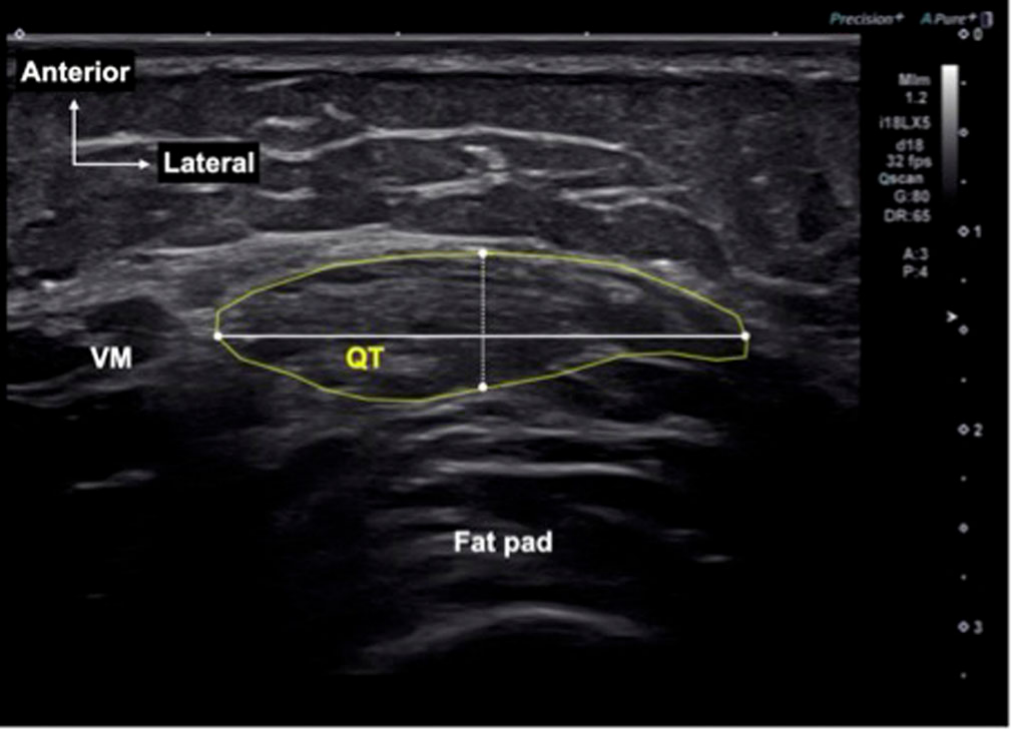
Measurement of anterior‐posterior thickness, medial‐lateral width, and cross‐sectional area (CSA) of the quadriceps tendon (QT) on magnetic resonance imaging. CSA (yellow line) was also measured at 15, 30, 45 and 60 mm proximal to the superior pole of the patella on short‐axis images. The widest medial‐lateral width (white line) was measured. The anterior‐posterior thickness (dotted line) was measured at the midpoint of the medial‐lateral width. VM, vastus medialis.
